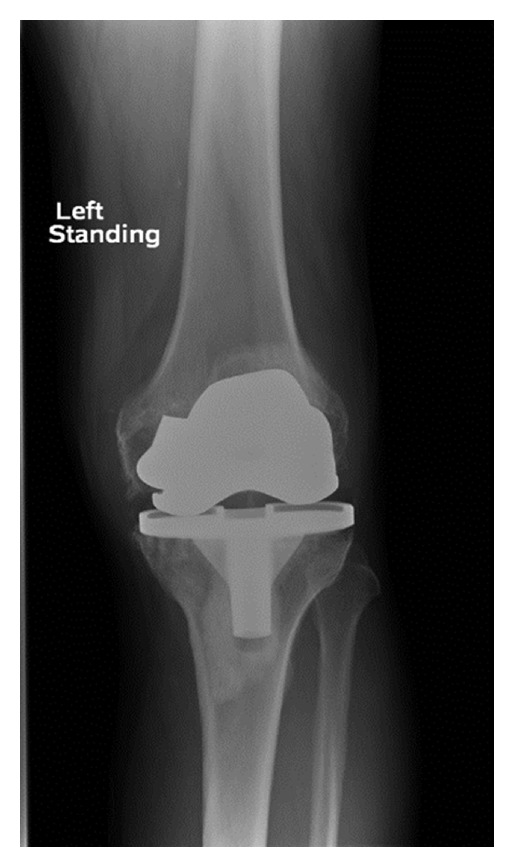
An anteroposterior radiograph of the knee showing evidence of the fracture of the medial femoral condylar prosthesis.
